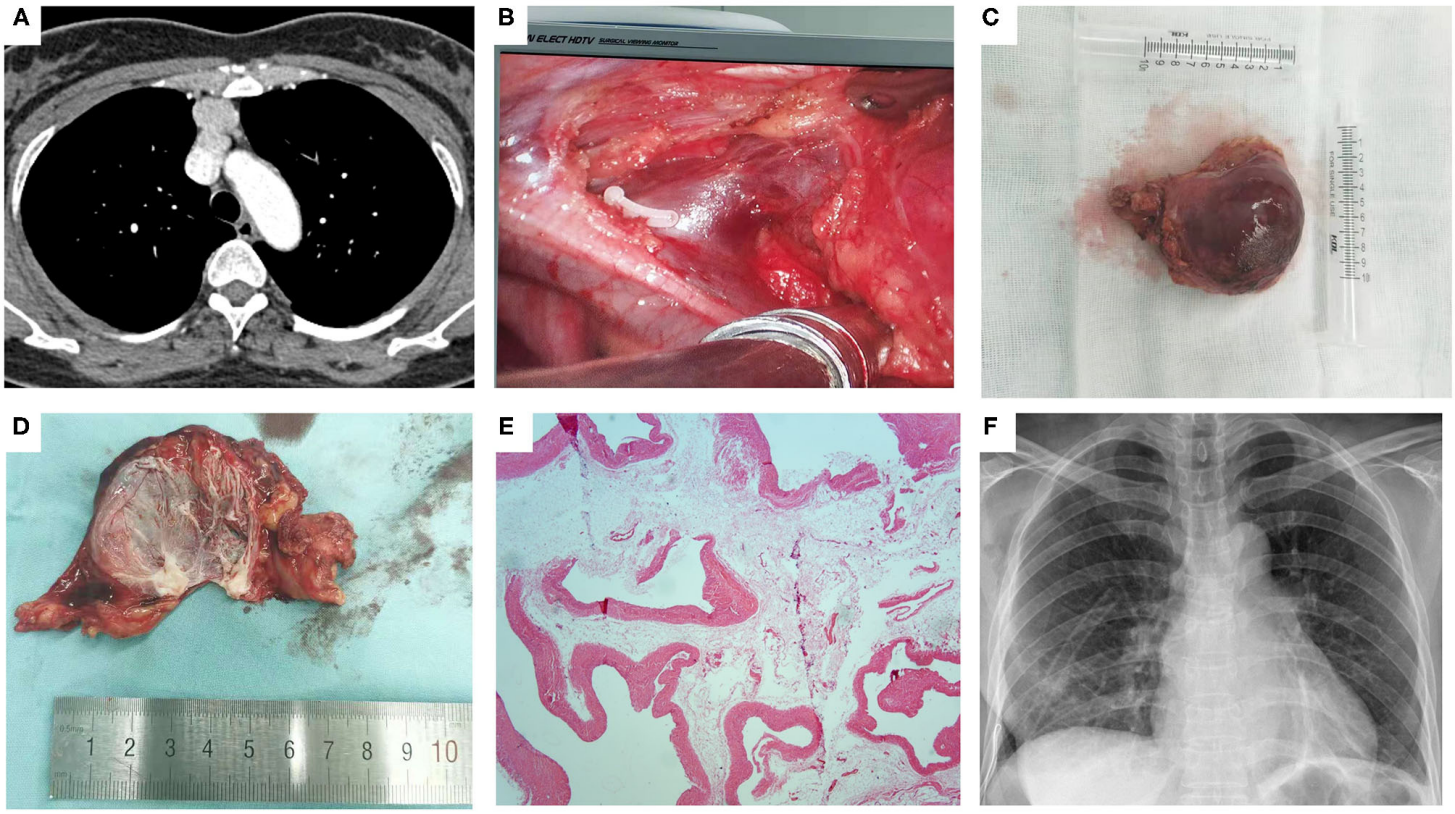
The left innominate vein aneurysm. (A) Contrast-enhanced CT of the chest showed a 4.8 × 4.6 cm anterior mediastinal mass with significant homogenous enhancement after injection of the contrast; (B) Intraoperative view of the root of the aneurysm after dividing the left internal thoracic vein with a vascular clamp; (C) Gross appearance of the aneurysm; (D) Gross appearance of the cut-up aneurysm after the blood being flowed away; (E) Postoperative pathology confirmed the diagnosis of the left innominate vein aneurysm; (F) Chest radiography on postoperative day 1 showed complete reexpansion of the lung, with no obvious pneumothorax or hydrothorax.
